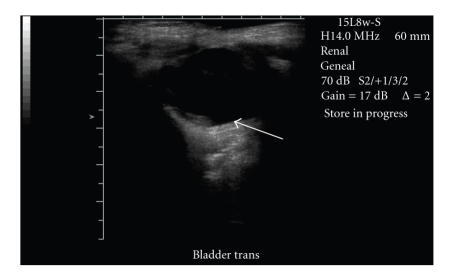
Ultrasound of pelvis of twin B at 2-month followup showing intact abdominal wall with full bladder (arrow).
